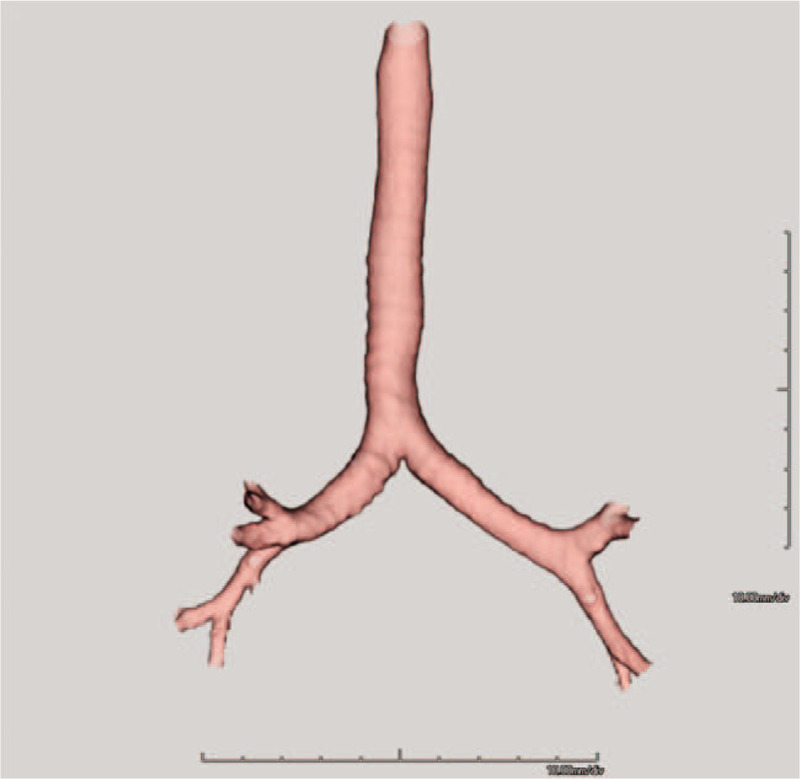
A 3-dimensional reconstructed computed tomography image for an asymptomatic, 36-yr-old man with incidentally detected isolated left bronchial isomerism. The image shows identical, long left main bronchi on the right and left sides and the absence of a right upper lobe bronchus.
